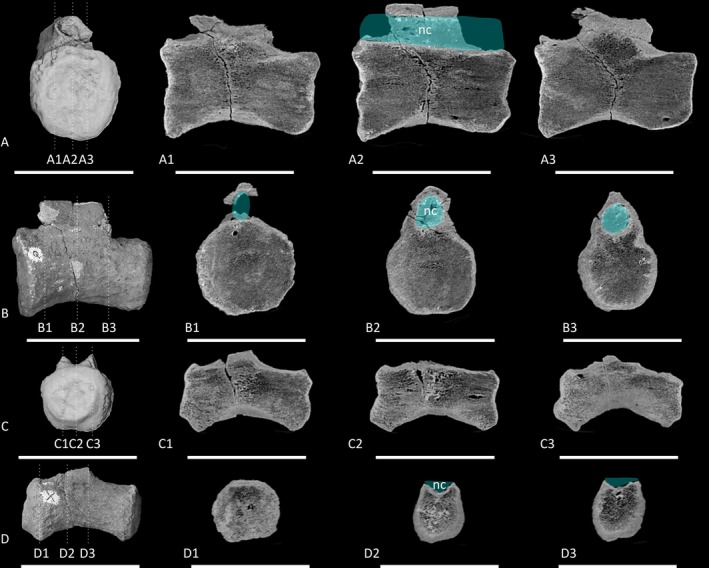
CT scan of *Wintonotitan wattsi* posterior caudal vertebrae QMF7292. (A, C) 3D rendering anterior view, (B, D) photograph left lateral view; (A, B) posterior caudal vertebra ‘Q’, (C, D) posterior caudal vertebra ‘X’; (A1–A3, C1–C3) CT scan slices in parasagittal cross‐section, (B1–B3, D1–D3) CT scan slices in transverse cross‐section. Nc, neural canal. Key: Blue highlight, neural canal. Scale bars = 100 mm.
